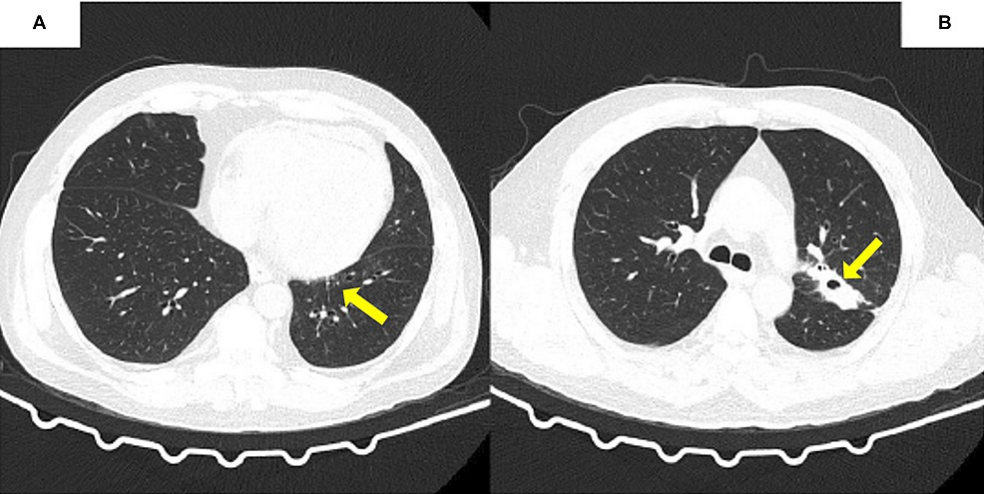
High-resolution computed tomography scan of the chest showing consolidation (A) and an excavated nodule, with thickened walls and inverted halo sign (B).
